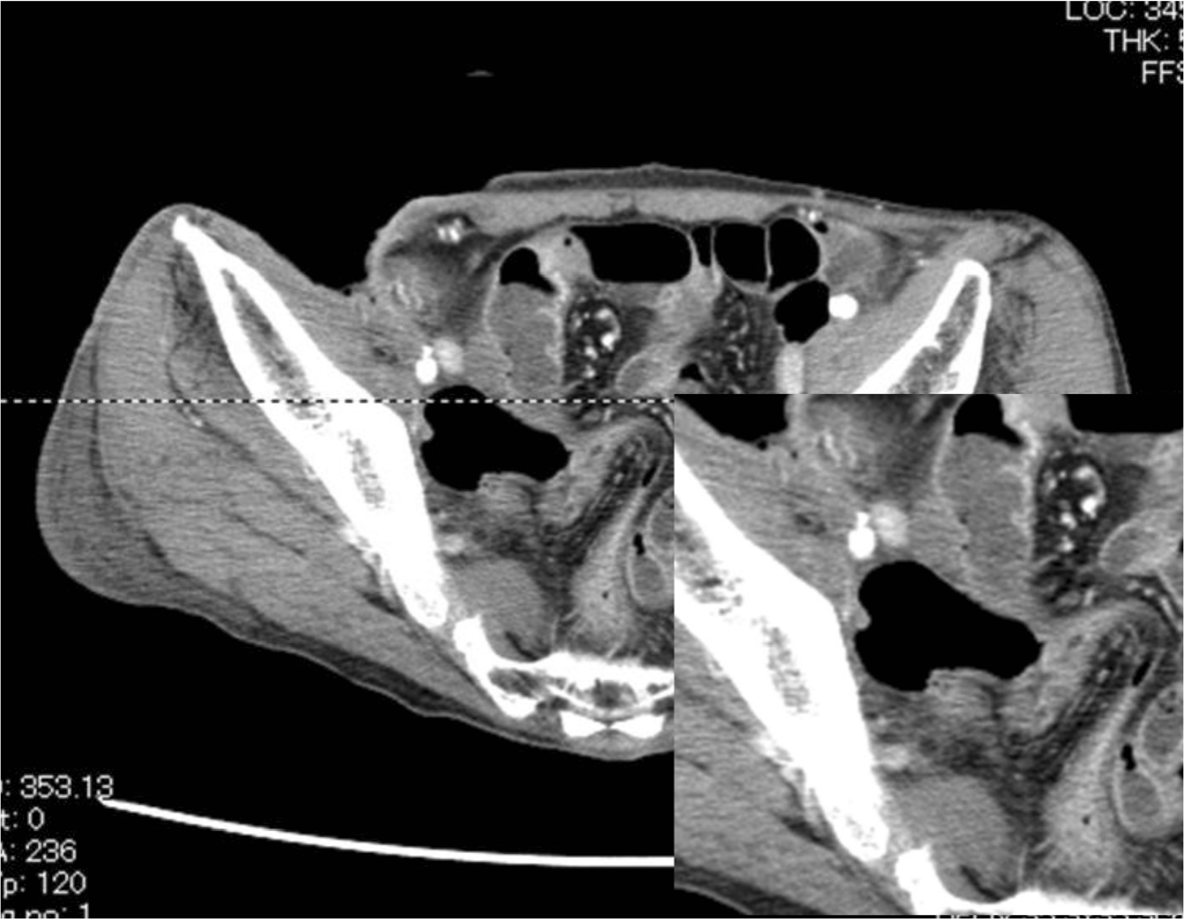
CT findings of the pelvis; CT showed the pseudoaneurysm of external iliac artery.
